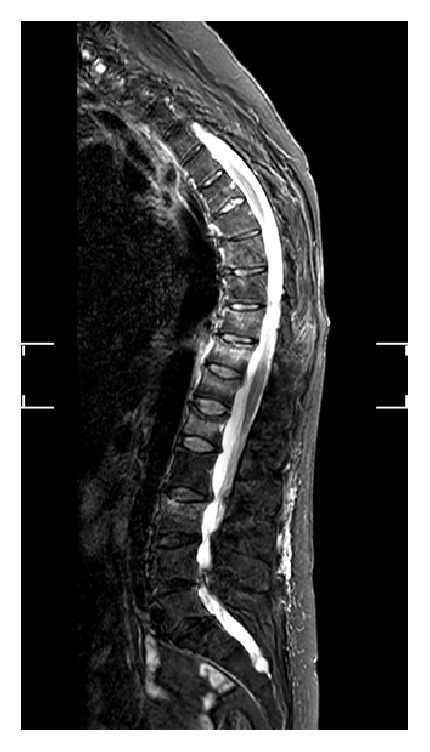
Preoperative sagittal magnetic resonance image (STIR sequence) of the lumbar spine showing edema of multiple vertebral bodies.
